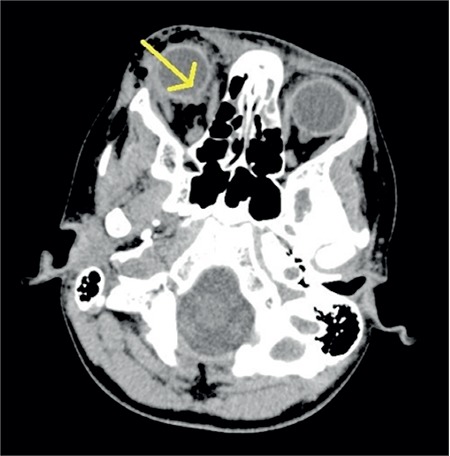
Computed tomography image taken one day after the trauma showing discontinuity of the right optic nerve in the sclera at the point of entry to the globe
